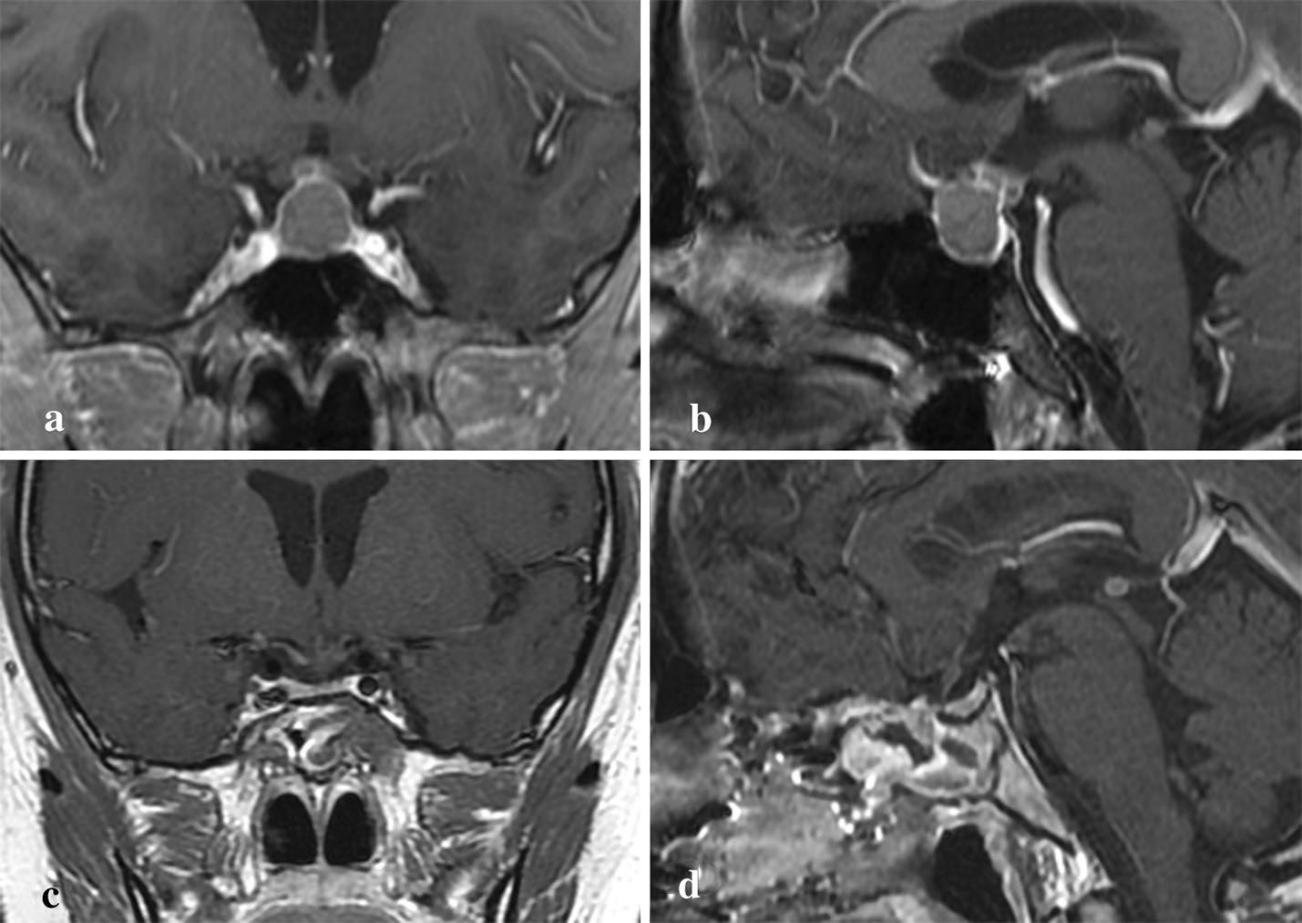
Case 1. a Preoperative coronal T1-weighted MR image with gadolinium revealing a large cystic lesion extending from the sella turcica to suprasellar cistern. b Preoperative sagittal T1-weighted MR image with gadolinium revealing than this cyst was multicystic. Cysts were collapsed after the operation (c, d)
